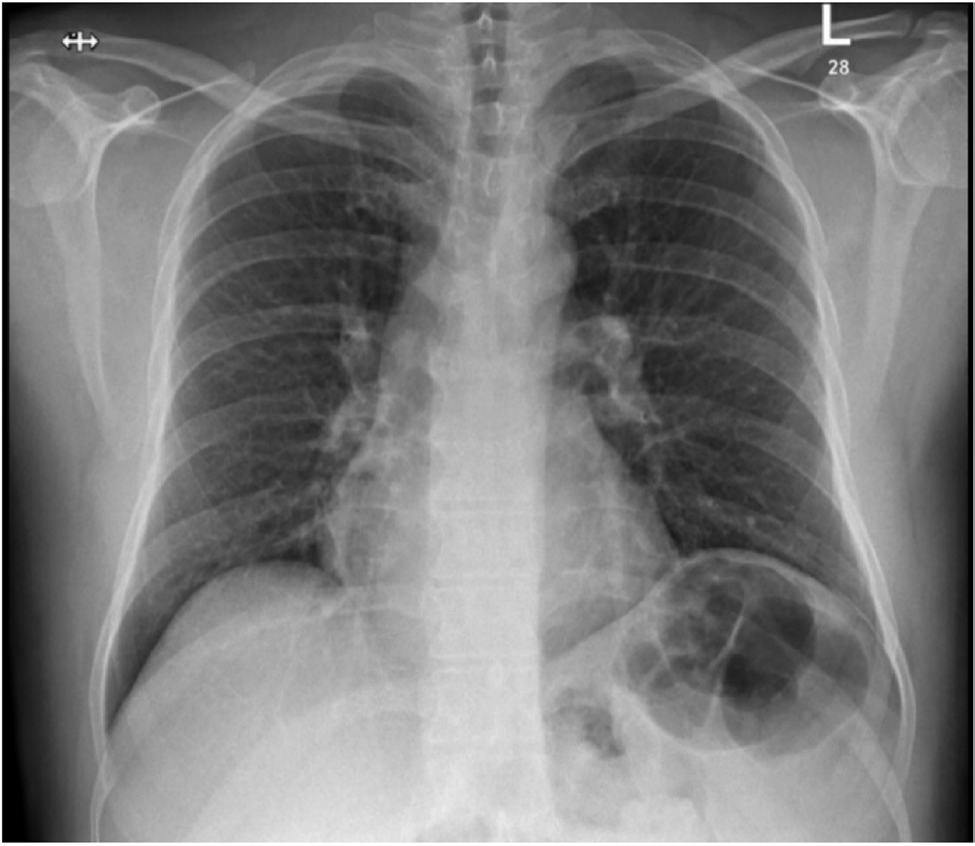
Chest computed tomography. No focal active lung lesion is seen. The heart is not enlarged. Both cardiophrenic angles are clear.
